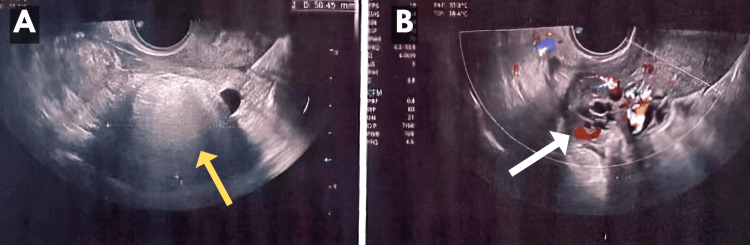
Ultrasonography findings A. Well-defined heterogeneously hyperechoic lesion of size 50x38 mm (yellow arrow); B. Right ovarian pedicle showing increased vascularity (white arrow)
